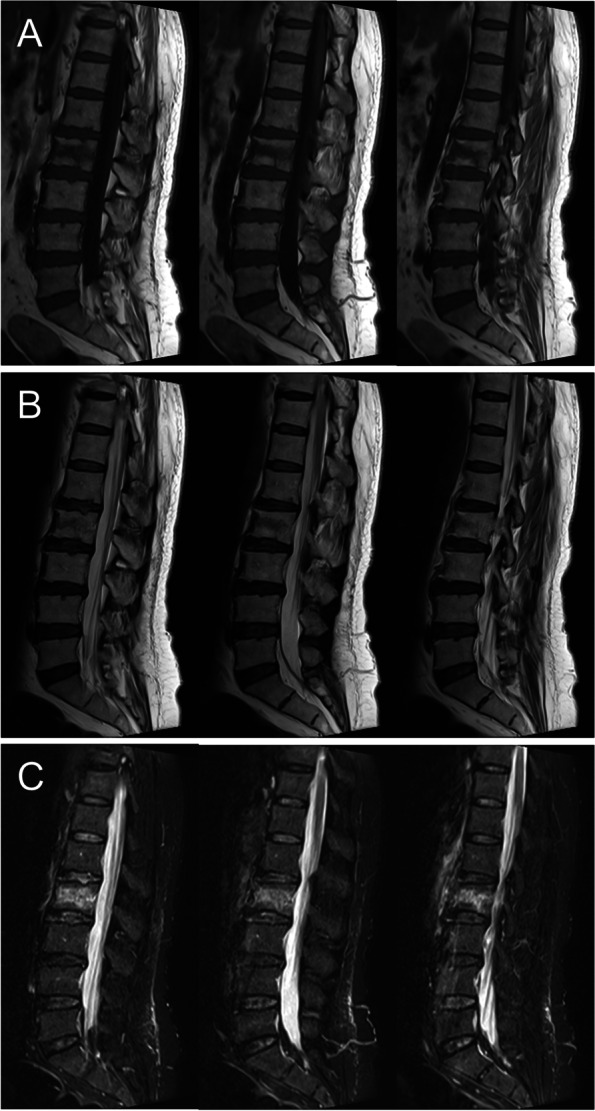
Osteoporotic vertebral fracture with non-severe fascia injury. Three most central slices of sagittal T1-weighted (A), T2-weighted (B) and T2-weighted fat suppression (C) MR images show L2 vertebral fracture with non-severe FI
